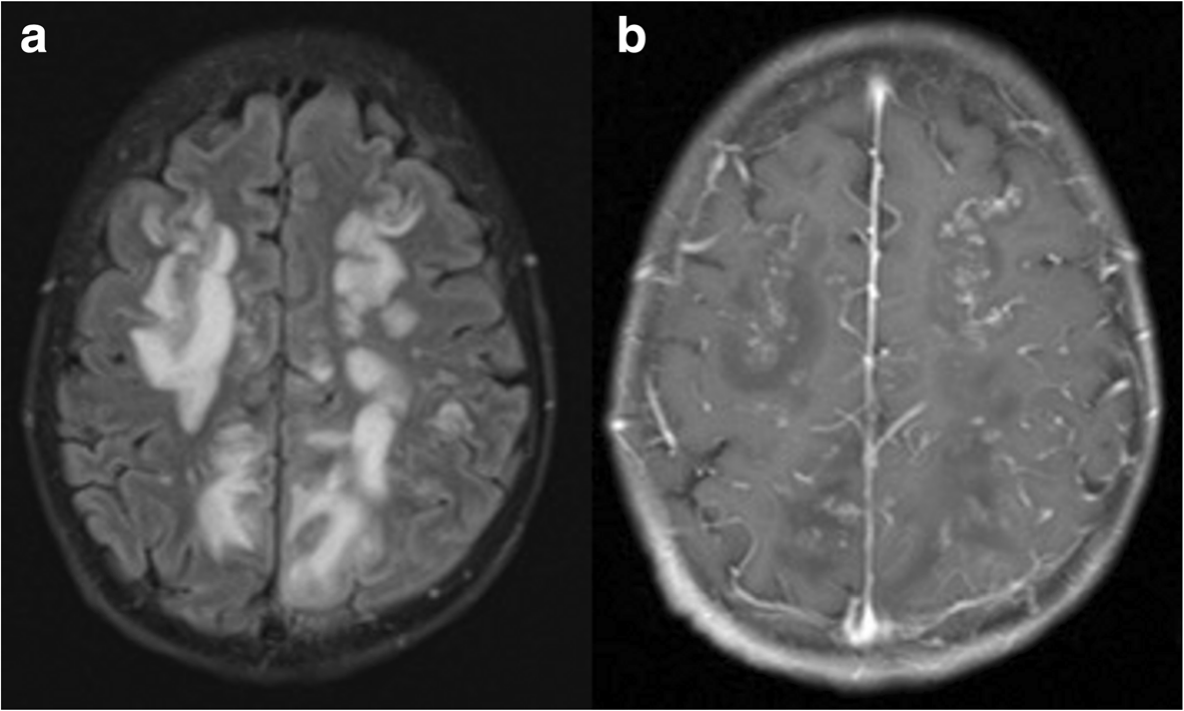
a and b Follow-up magnetic resonance imaging (MRI) of the brain. Follow-up MRI of the brain 3 days after the first scan demonstrated a significant increase in size and extension of the now-confluent white matter lesions on the axial 3-mm fluid-attenuated inversion recovery image (a), especially notable bilaterally in the frontal and parietal lobes with stipple contrast enhancement with a “miliar” distribution pattern (b; axial T1-weighted image after contrast application, 3 mm)
